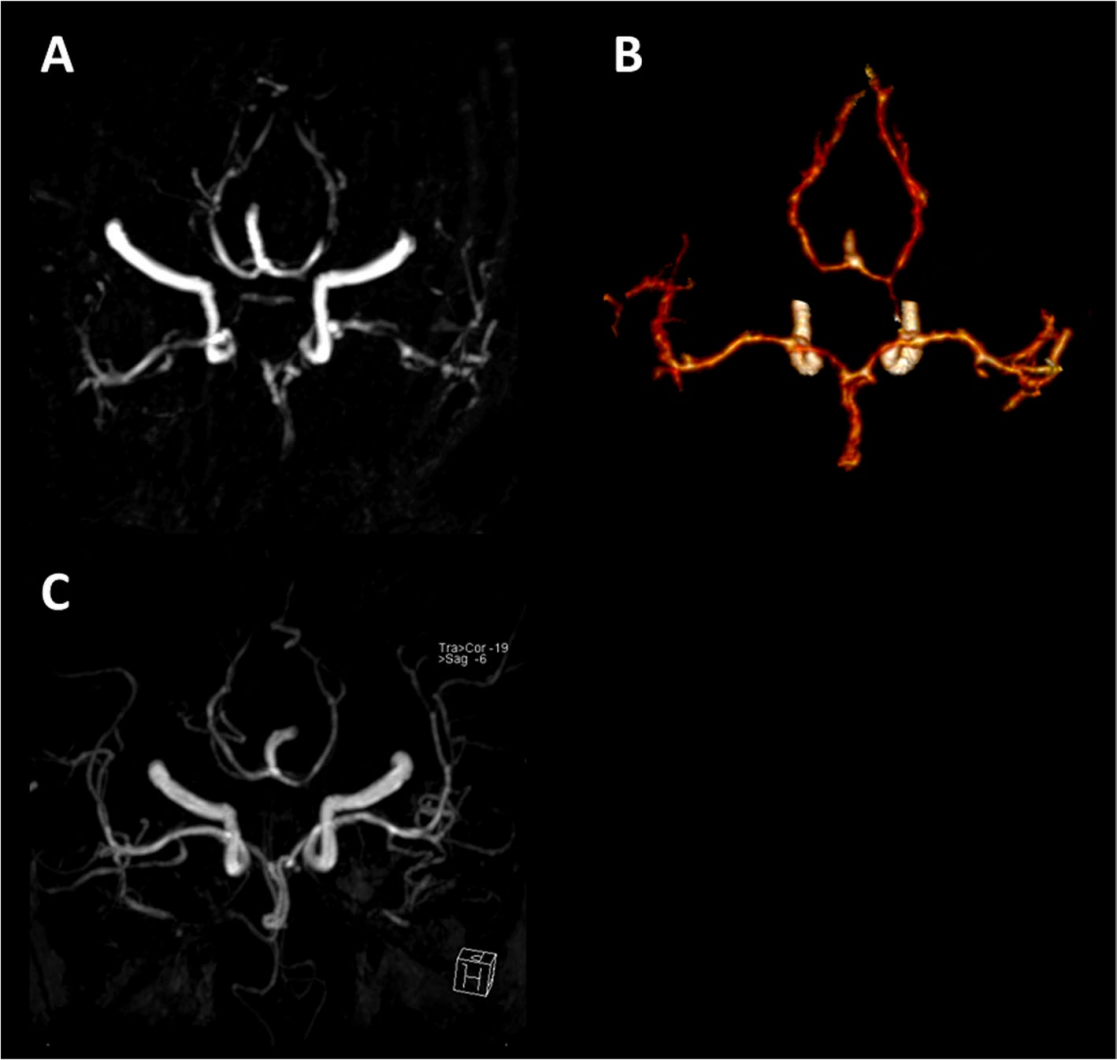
Initial and follow-up CTA and MRA. The 15 days and 21 days after ethylene oxide inhalation, Head MRA (A) and CTA (B) showed multiple areas of stenosis and partial occlusions involving the anterior, medial and posterior cerebral arteries bilaterally, respectively. The 59 days after ethylene oxide inhalation, the follow-up head MRA (C) showed that the cerebral arterial stenosis was markedly improved but not complete recovery
